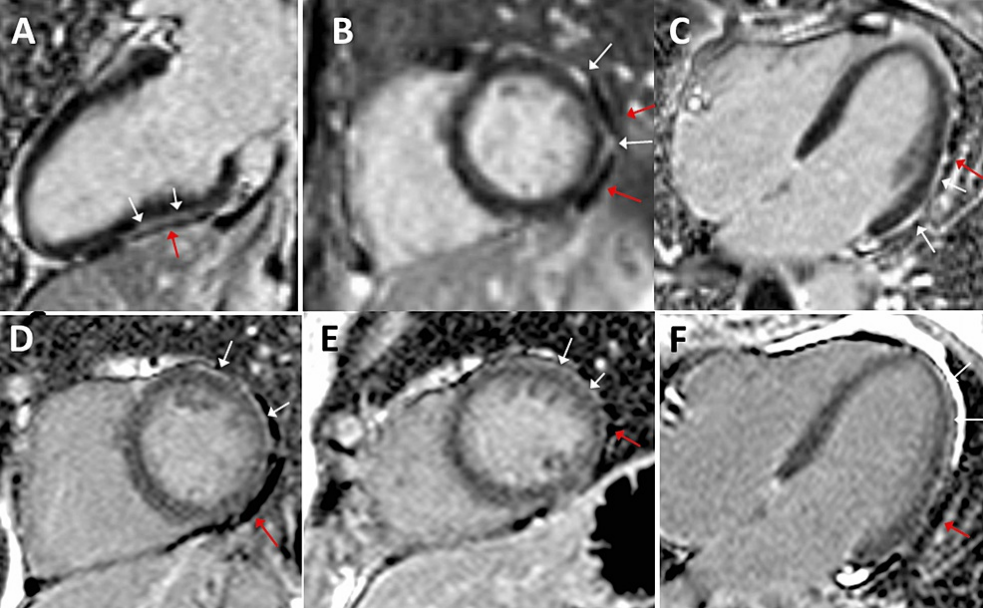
Cardiac MRI in June 2022 (panels A-C) showing late gadolinium enhancement in the basal, apical, anterolateral, and inferolateral segments (white arrows) and pericardial effusion (red arrows). These abnormalities were still present on a follow-up MRI in October 2023 (panels D-F).
